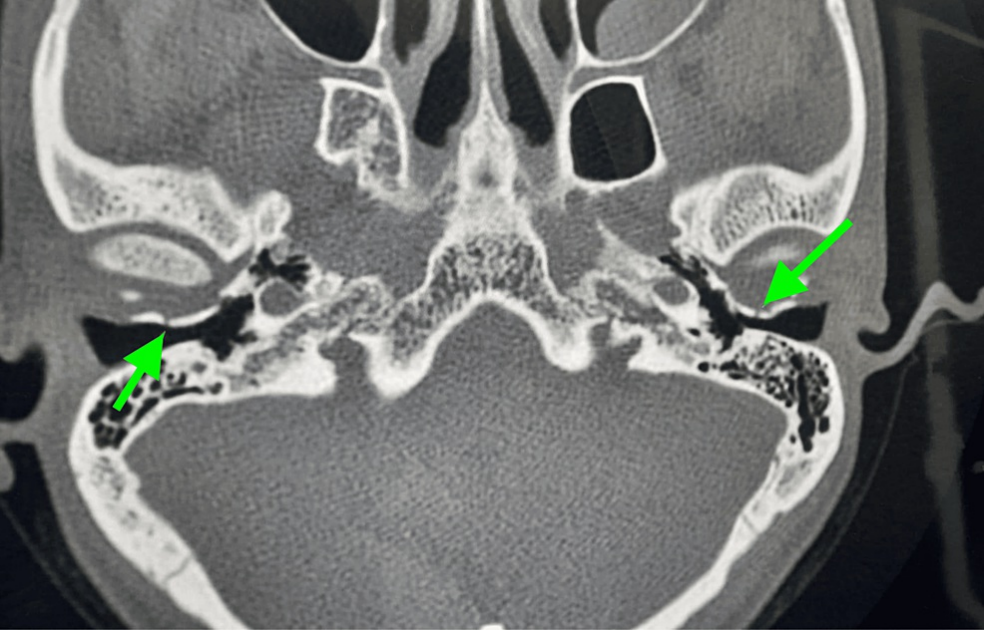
An HRCT axial cut showing bilateral undisplaced linear fractures (green arrows) of the anterior wall of the bony part of the bilateral external auditory canal (tympanic part). HRCT: high-resolution computed tomography
